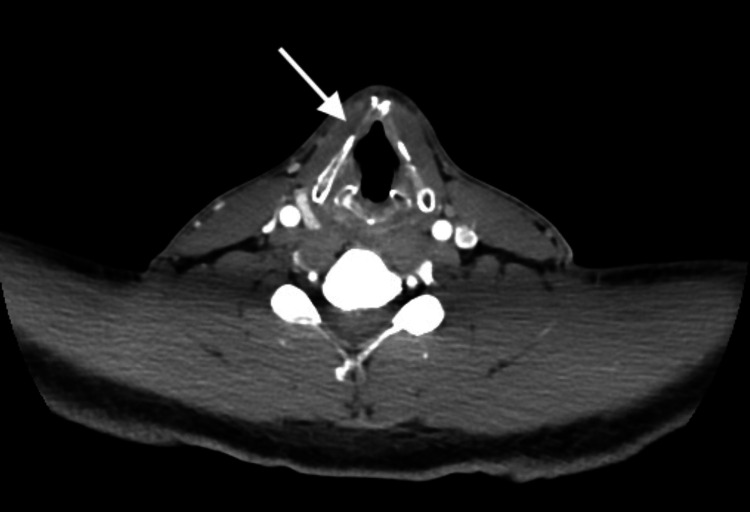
CT of the neck with contrast showing right vocal cord medialization
